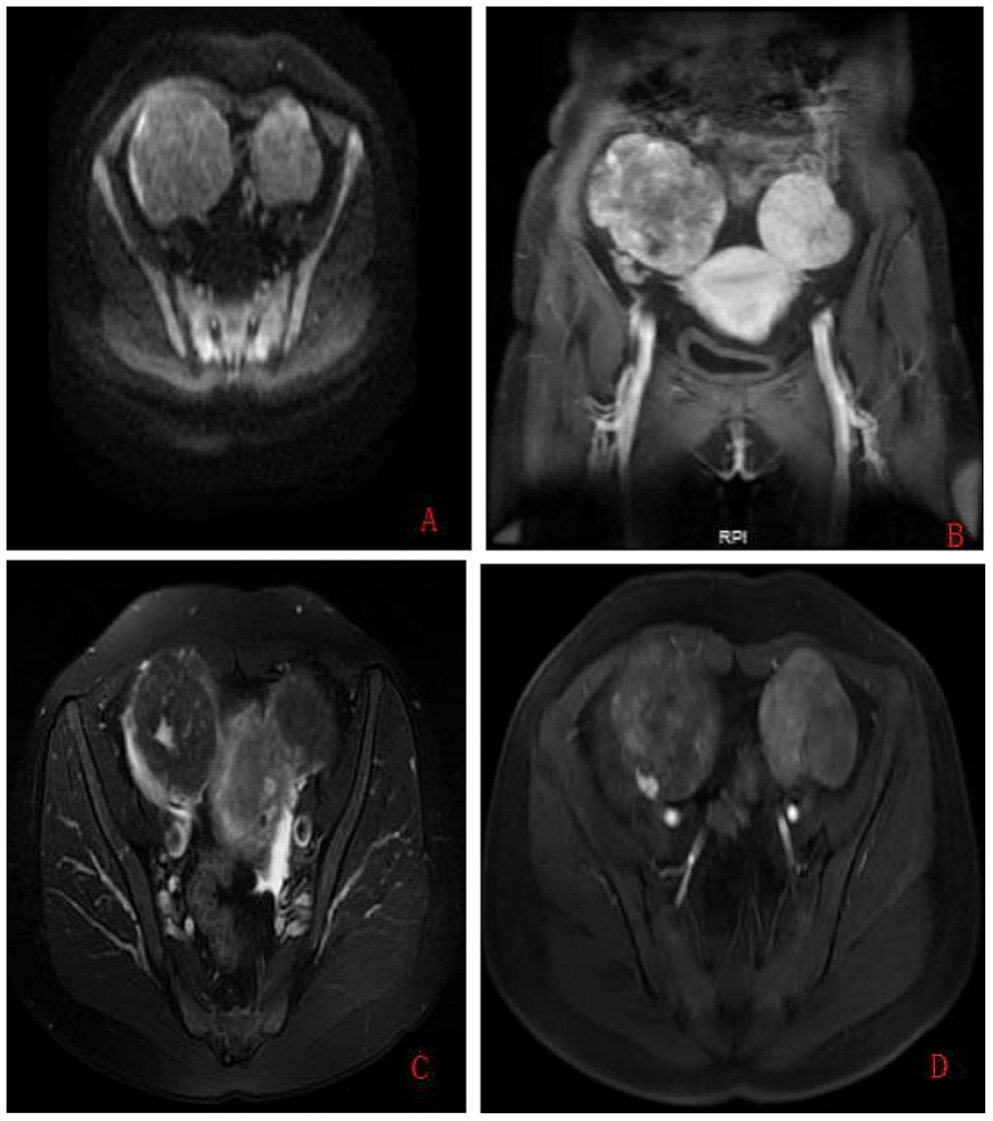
Preoperative pelvic MRI findings obtained for case 8. (A) On axial diffusion-weighted image (repetition time (TR)/echo time (TE): 4,000/72.4 ms, *b*-value = 500 s/mm^2^); the two masses showed the same intermediate signal intensity. (B) On sagittal T1-weighted with gadolinium administration (TR/TE: 3.9/1.7 ms), the left mass showed low signal intensity identical to the uterus myometrium. (C) On axial fat-saturated T2-weighted (TR/TE: 3,020/70.5 ms) image, the signal intensity of the left mass was continuous with the myometrium, while the right mass was well-circumscribed and sharply demarcated from the uterus. (D) On axial T1-weighted with gadolinium administration (TR/TE: 3.9/1.7 ms), flow voids were visible only between the tumor and the uterus myometrium on the left mass.
